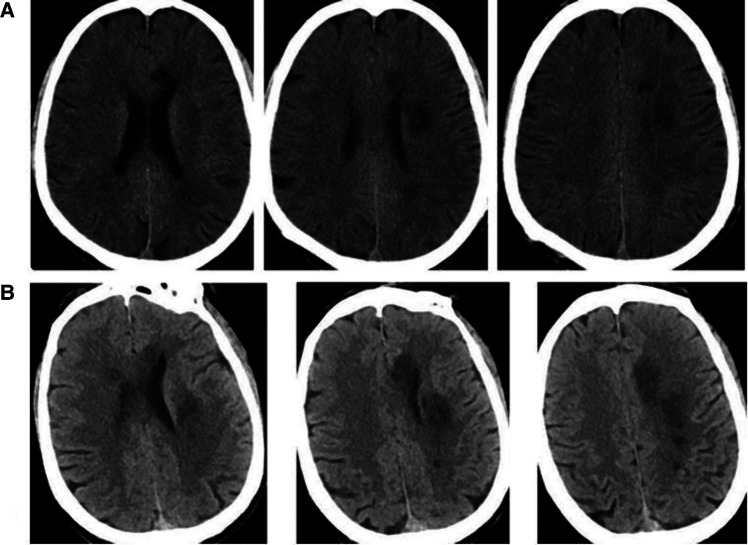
(A) Brain CT images were reexamined on the day after CAS. (B) On the third day after CAS, the brain CT image was reexamined (the low-density focus showing a greater enlargement than before). CAS, carotid artery stent implantation.
